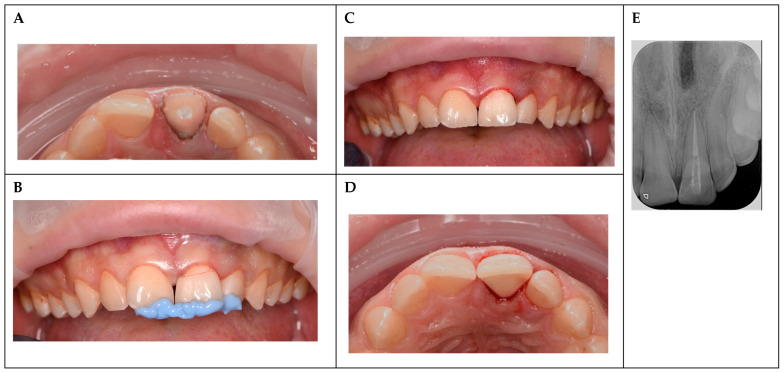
Treatment procedures. (A) Occlusal view after isolation. (B) Buccal view of the check-up fit of the placed fragment, with a liquid rubber dam guide in place. (C) Buccal view of the reattached fragment and restored tooth. (D) Occlusal view of the reattached fragment and restored tooth. (E) Periapical radiograph post-endodontic treatment and fiber-post placement, demonstrating hermetic root canal obturation and post stability.
